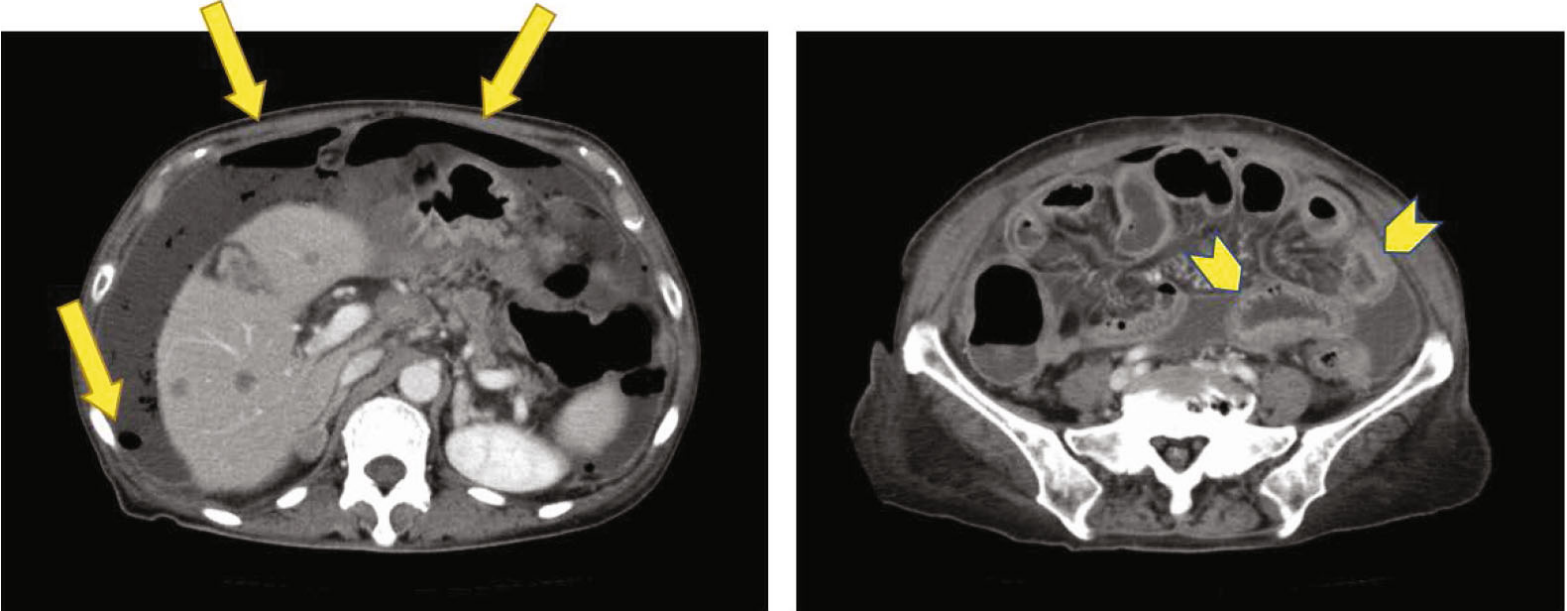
The abdominal CT findings. Abdominal CT showed free air (single arrow) and thickening of the bowel wall (arrowhead).
